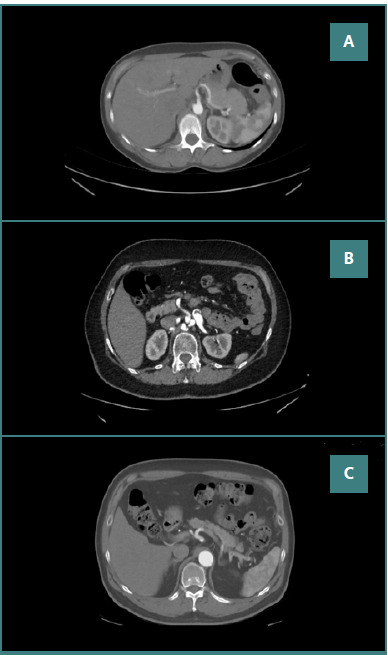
CTA imaging of celiac ganglia positions in relation to celiac trunk variants. A, Hepatogastric trunk, splenic artery from superior mesenteric artery, the celiac ganglion is posterolateral to the celiac trunk; B, Hepatosplenic trunk, left gastric artery from abdominal aorta, the celiac ganglion is posterior to the celiac trunk; C, Hepatosplenic trunk, left gastric artery from splenic artery, the celiac ganglion is inferior to celiac trunk.
